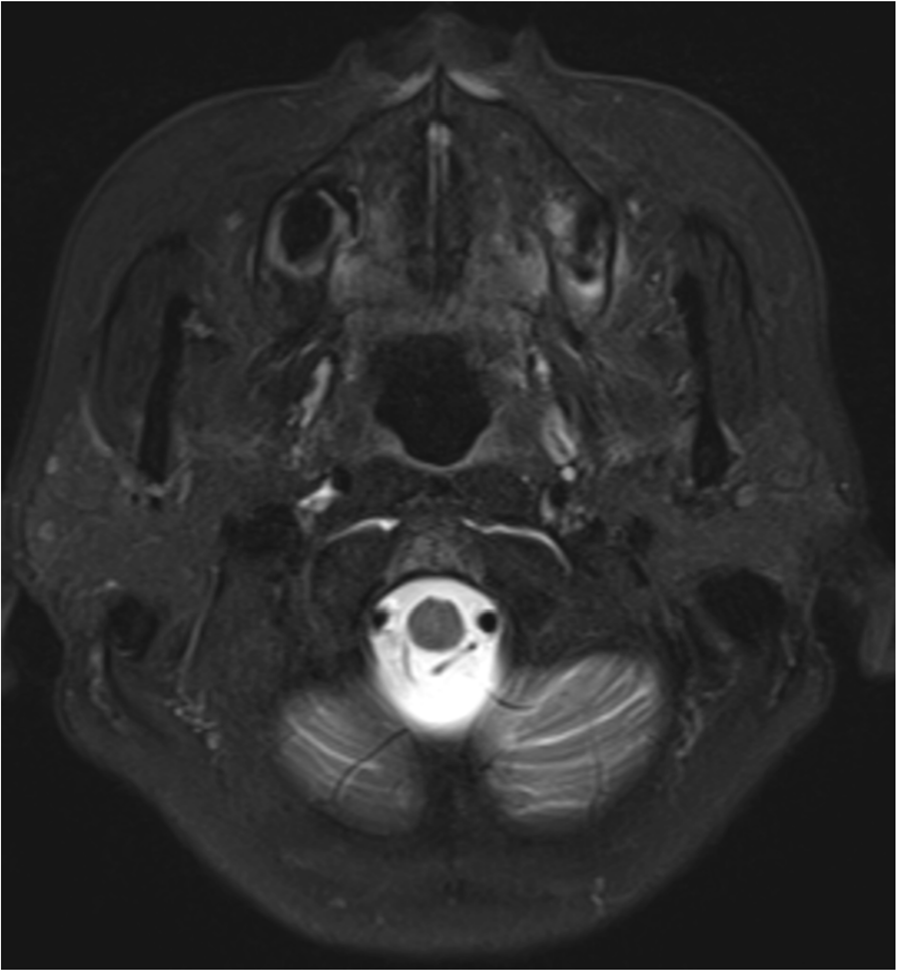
Axial fat-suppressed T2-weighted MR image showing that the primary tumor is not detected in the mandibular ramus and pterygoid region
